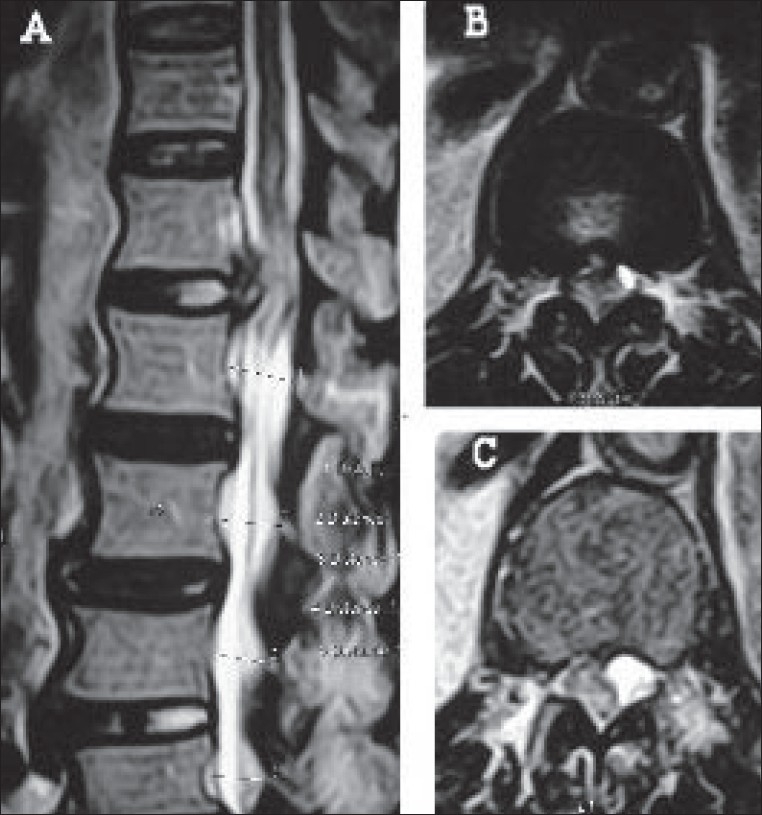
T2W MRI sagittal (A) and axial images (B and C) showing disc herniation at D12-L1. Disc fragment lying beneath the D12 lamina displacing conus antero-laterally and cord edema (C)
